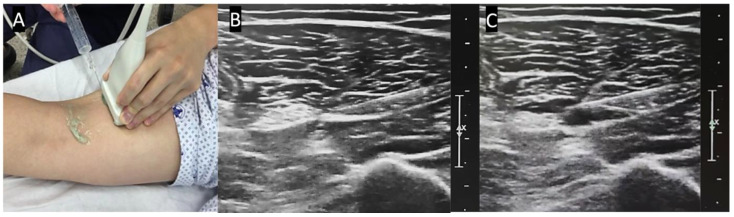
The popliteal block was performed under real-time ultrasound guidance. (A) From the popliteal crease, the tibial nerve was traced proximally along the posterior thigh. (B) The target of injection was chosen just proximal to the bifurcation point of the sciatic nerve. A needle was placed with a short-axis, in-plane, and lateral side approach to the sciatic nerve. (C) After confirming the needle tip position, subparaneural injection was performed with 15 mL of 1% lidocaine.
